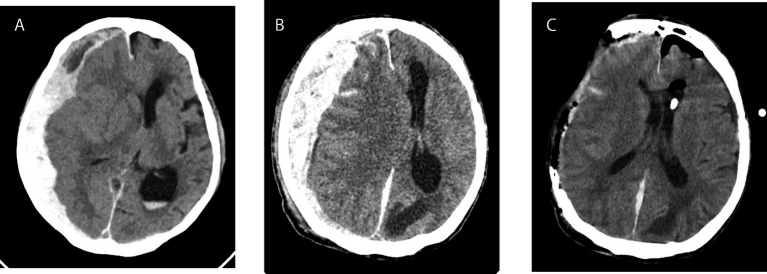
Initial axial brain CT scan showing acute subdural hematoma (aSDH) of the right cerebral hemisphere, compression of the right lateral and third ventricle, subfalcine and uncal herniation, and midline shift to the left for 1.7 cm. A considerable amount of blood is seen in the enlarged temporal horn of the left lateral ventricle (A). Initial native axial brain CT scan of another patient showing a large peri-hemispheric right-sided SDH with compressive mass effect and a substantial midline shift, displacing the supratentorial ventricles contralaterally. Initial ventricular dilatation with enlargement of the left lateral ventricle’s temporal horn is also visible (B). Post-operative CT scan of the same patient depicting resolution of aSDH and mass effect, slight ventriculomegaly, and the tip of the EDV placed in the left lateral ventricle frontal horn (C).
